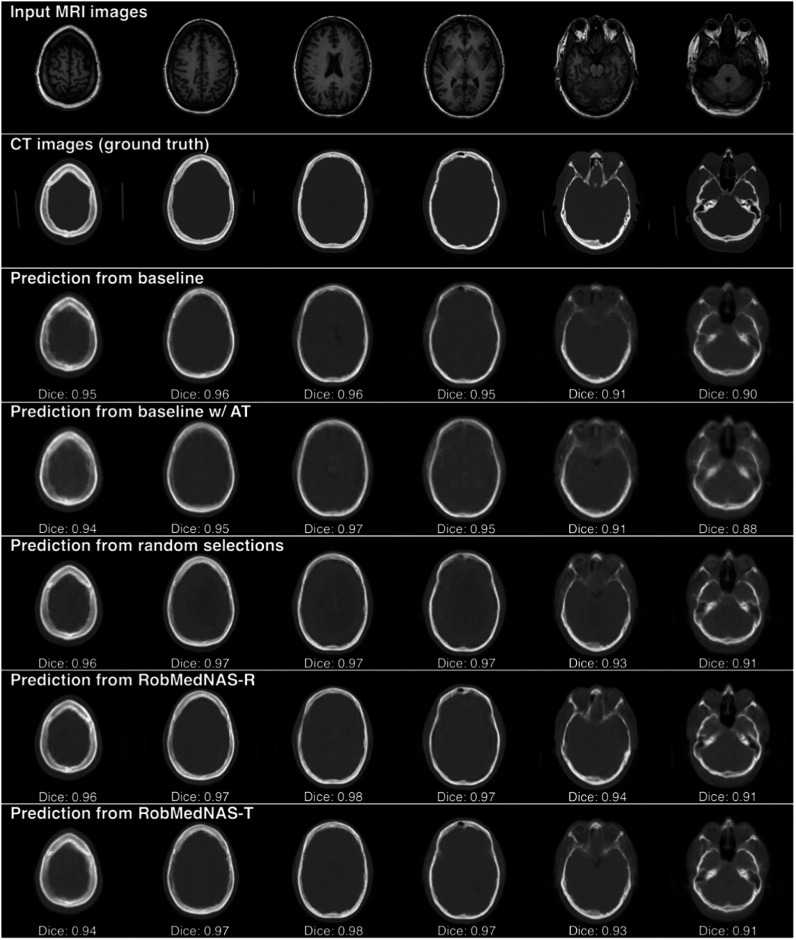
The performance of different models on selected MRI slices. We calculate the Dice coefficient values by averaging on the region of brain, air, bone and soft tissues.
